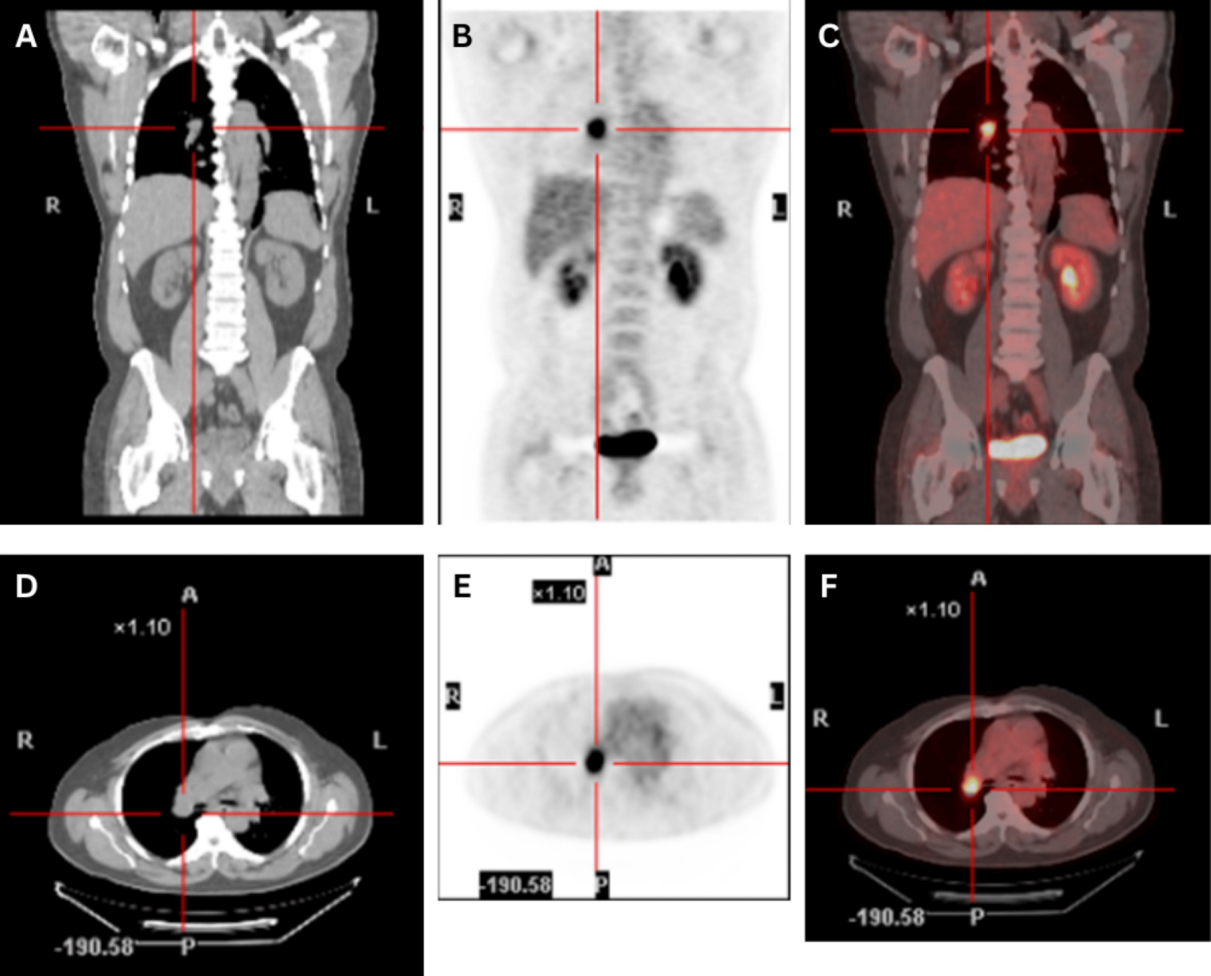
Intensely FDG-avid right hilar lymph node on PET/CT A: CT Coronal view. B: PET Coronal view. C: Fused Coronal view. D: CT Transaxial view. E: PET Transaxial view. F: Fused Transaxial view.
